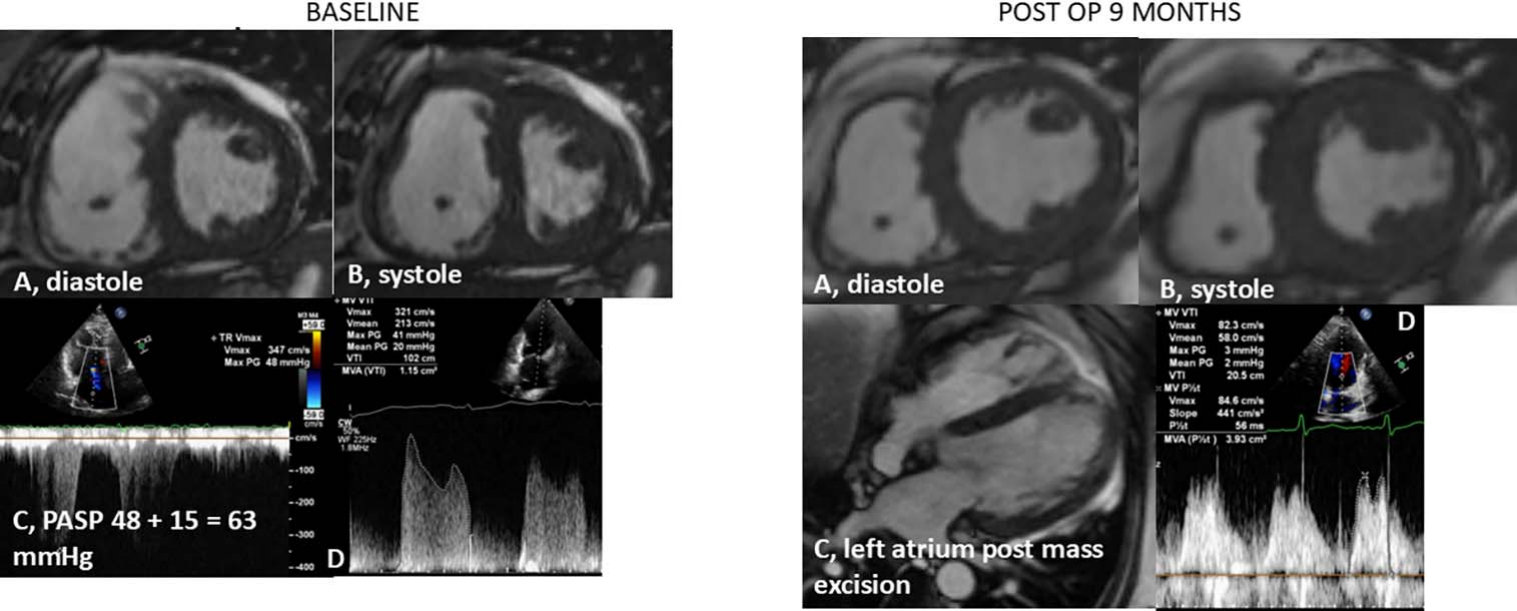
Left panel, baseline imaging: (A) Preoperative cardiac magnetic resonance imaging (MRI) in diastole. (B) Preoperative cardiac MRI in systole shows a D-shaped septum consistent with pulmonary hypertension from functional mitral stenosis. (C) Echocardiography shows pulmonary artery systolic pressure (PASP) of 48 mm Hg. (D) Echocardiography shows PASP elevated at 63 mm Hg, peak mitral inflow velocity of 321 cm/s, and mean gradient of 20 mm Hg. Right panel, postoperative imaging: (A) Postoperative cardiac MRI in diastole. (B) Postoperative cardiac MRI in systole shows resolution of the D-shaped septum. (C) Cardiac MRI of the left atrium after excision of the atrial masses. (D) Echocardiography shows postoperative normalization of the peak transmitral velocity and mean gradient.
